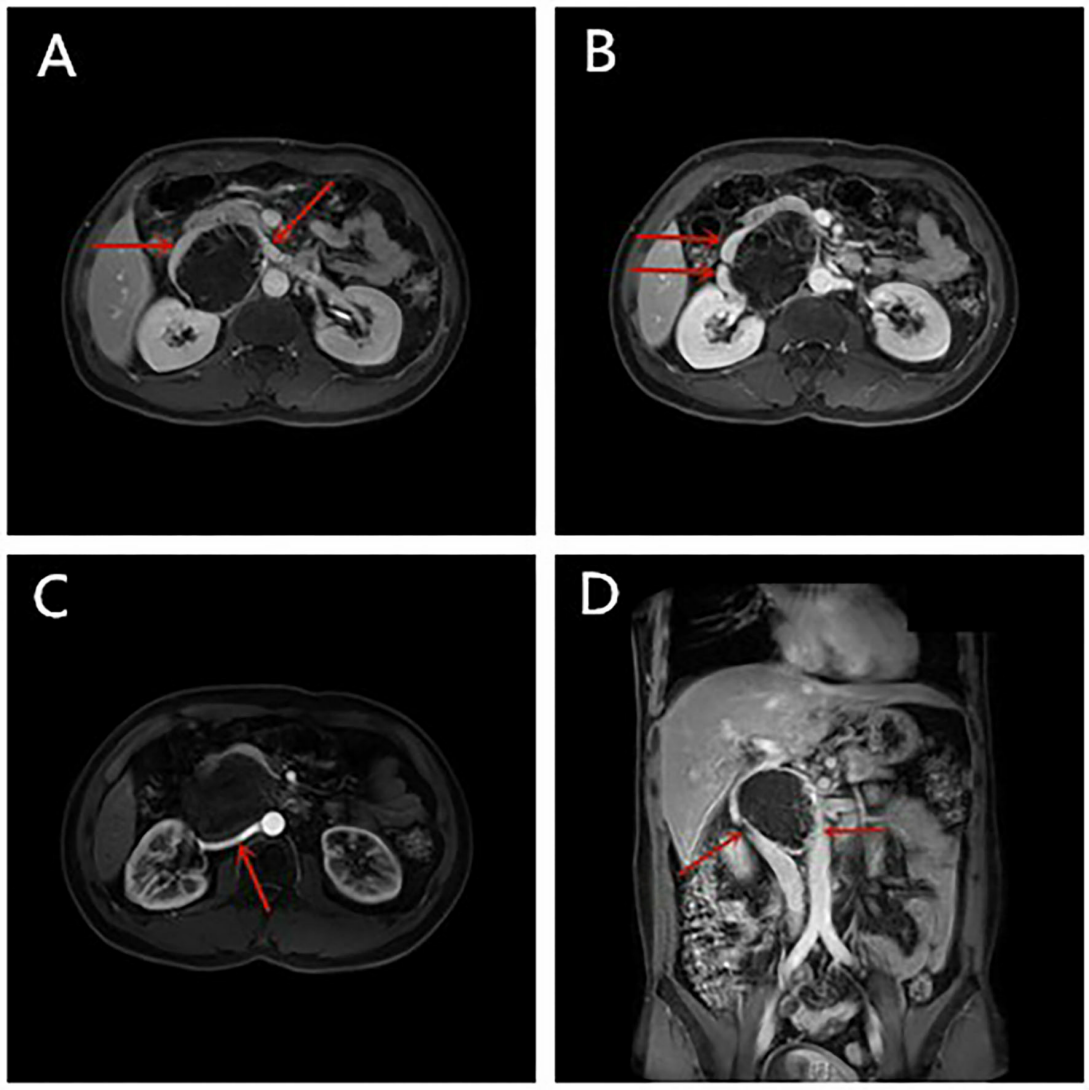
(A–D) The enhanced Magnetic Resonance Imaging (MRI) performance of Case 1.
